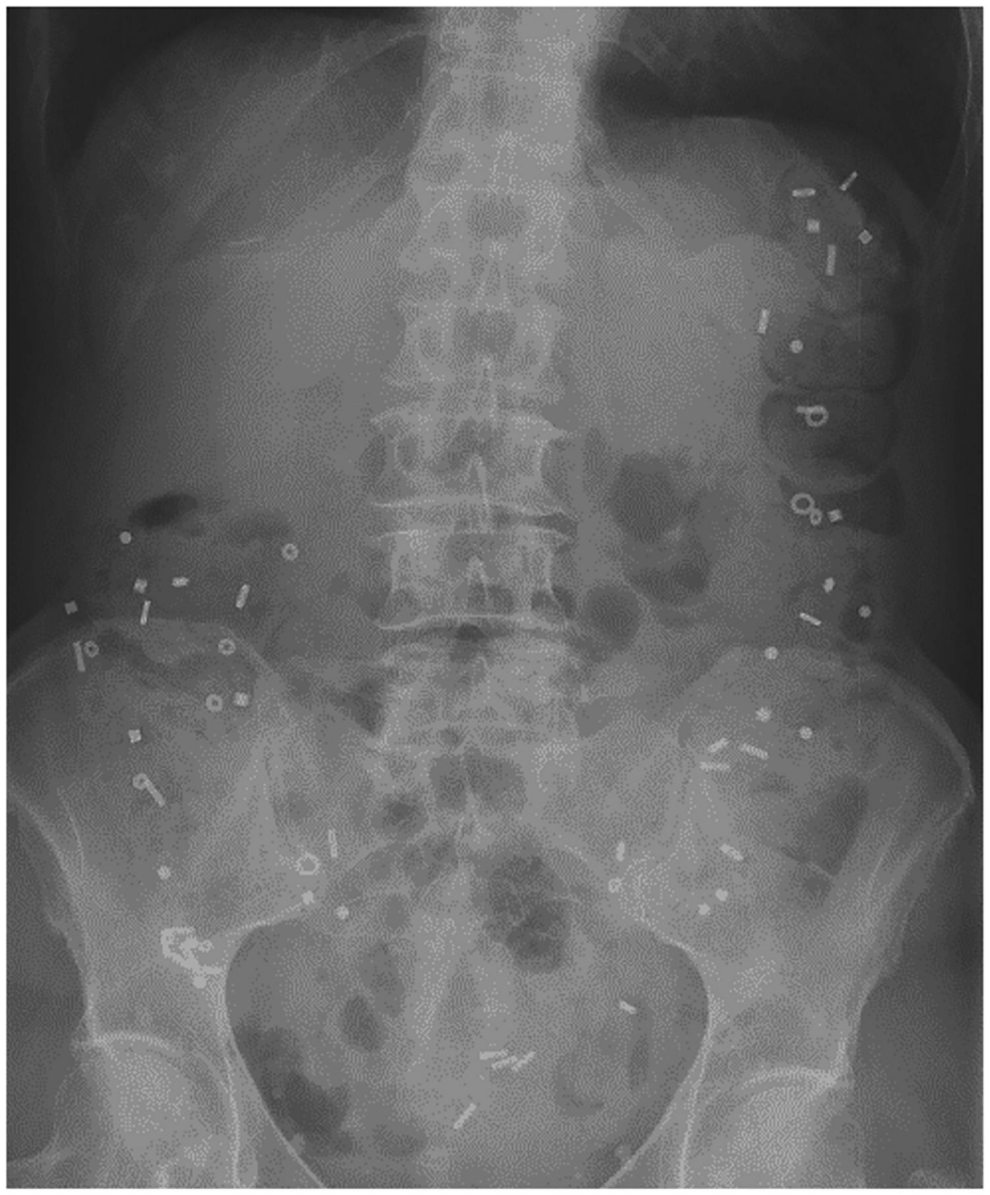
Assessment of colonic transit time with radiopaque markers. A radiopaque marker test of a patient who ingested 10 markers every morning for 6 days is shown. The plain abdominal radiograph was taken on day 7 and shows that all 60 markers are retained; accordingly, colonic transit time is ≥144 h ((number of retained capsules × 24 h)/(number of capsules ingested per day)). Normal values include colonic transit times ≤70 h in a mixed population, ≤50 h in men and ≤70–106 h in women. Note that in this case, the markers are evenly distributed throughout the colon, which is regarded as typical of, but is not completely specific for, slow-transit constipation.
